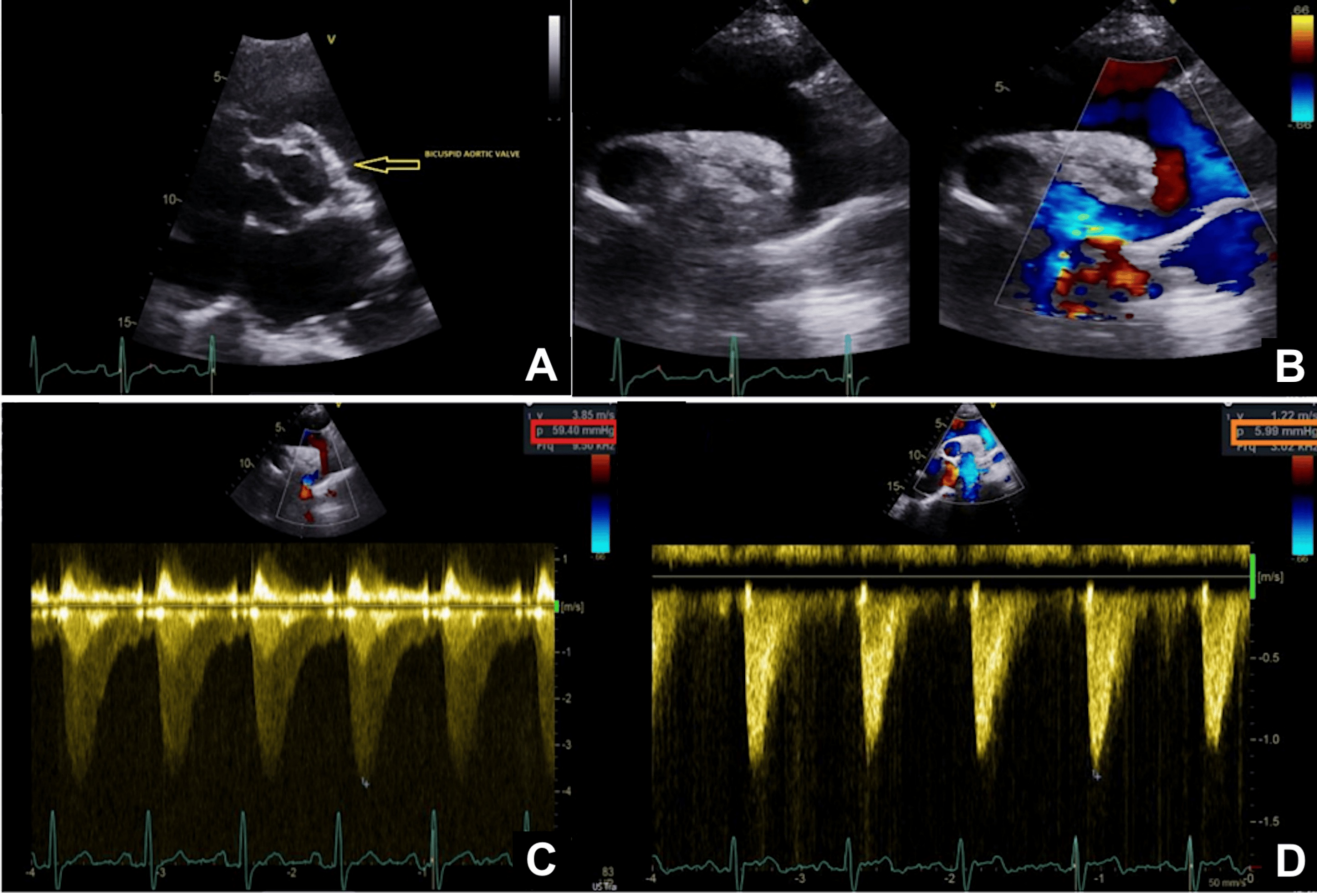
Transthoracic echocardiogram images (A) Bicuspid aortic valve (yellow arrow); (B) normal and colour flow across aortic arch showing turbulence; (C) pre-procedural gradients across coarctation; red box (59.4 mmHg); (D) post-procedural normalised gradients across the same segment; orange box (5.99 mmHg).
